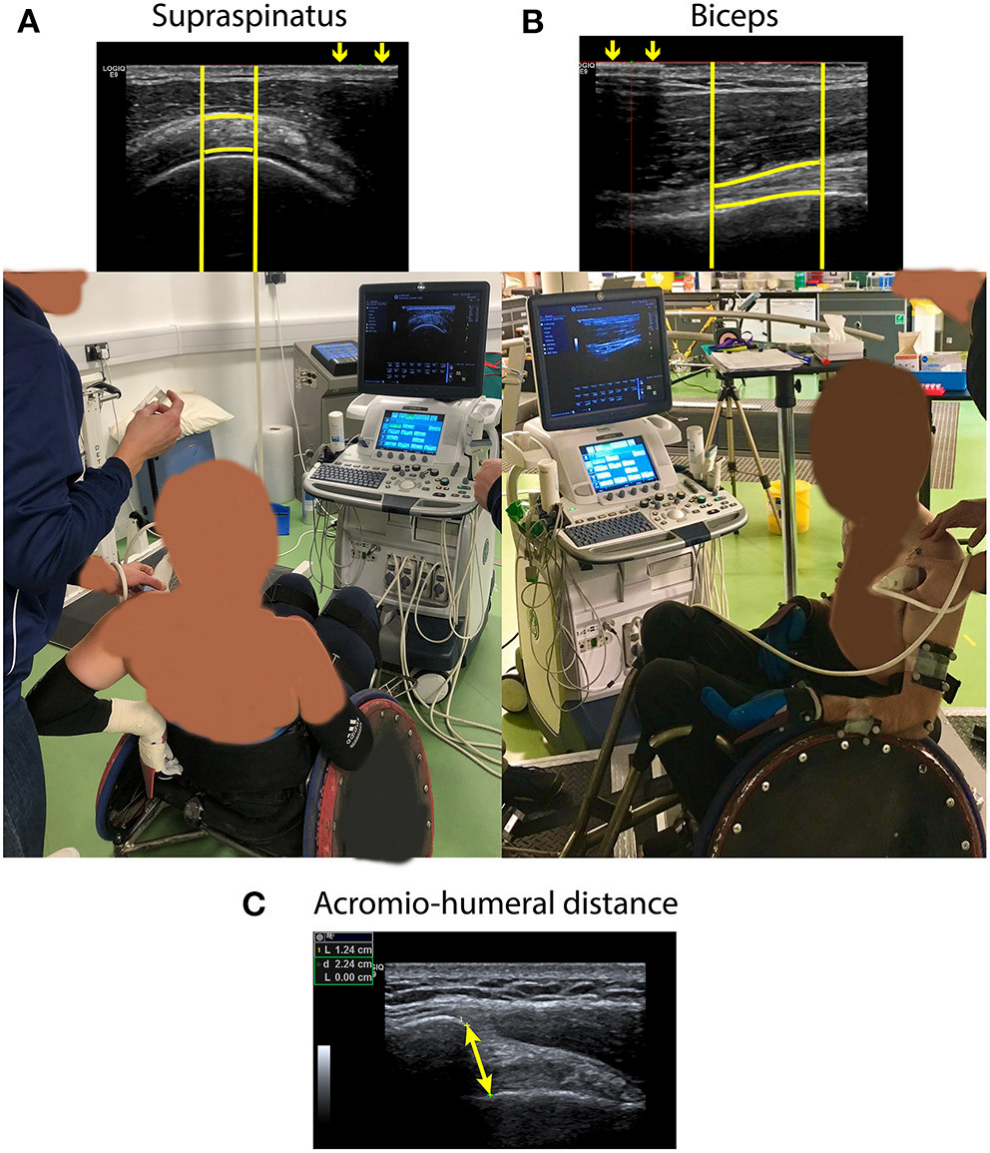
(A) Ultrasound image of supraspinatus tendon taken following Quantitative Ultrasound Protocols (QUS) and position in which the image was taken. (B) Ultrasound image of biceps tendon taken following QUS and position in which the image was taken. For both (A) and (B), arrows demonstrate the interference pattern that resulted from a metal marker taped to the skin from which the bright vertical lines were defined. Bright horizontal lines following the tendon borders are manually defined. (C) Ultrasound image of the acromio-humeral distance (i.e., the shortest distance between the anterior inferior edge of the acromion and the humeral head). The image was taken in the same position as the image of the biceps tendon, but with the thumbs facing upwards.
